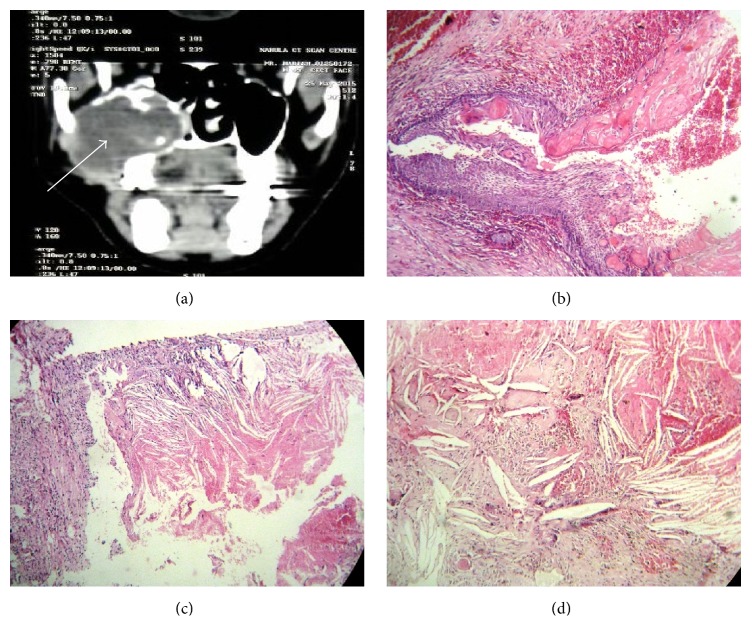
(a) CT scan image showing huge expansile, well demarcated, and osteolytic mass extending from maxilla into the maxillary sinus on right side; (b) proliferation of cystic odontogenic epithelial lining with palisaded hyperchromatic columnar nuclei, stellate reticulum like areas, and sheets of abundant ghost cells (H/E 4x); (c) cholesterol clefts in cystic wall (H/E 4x); (d) cholesterol clefts in cystic wall (H/E 10x).
